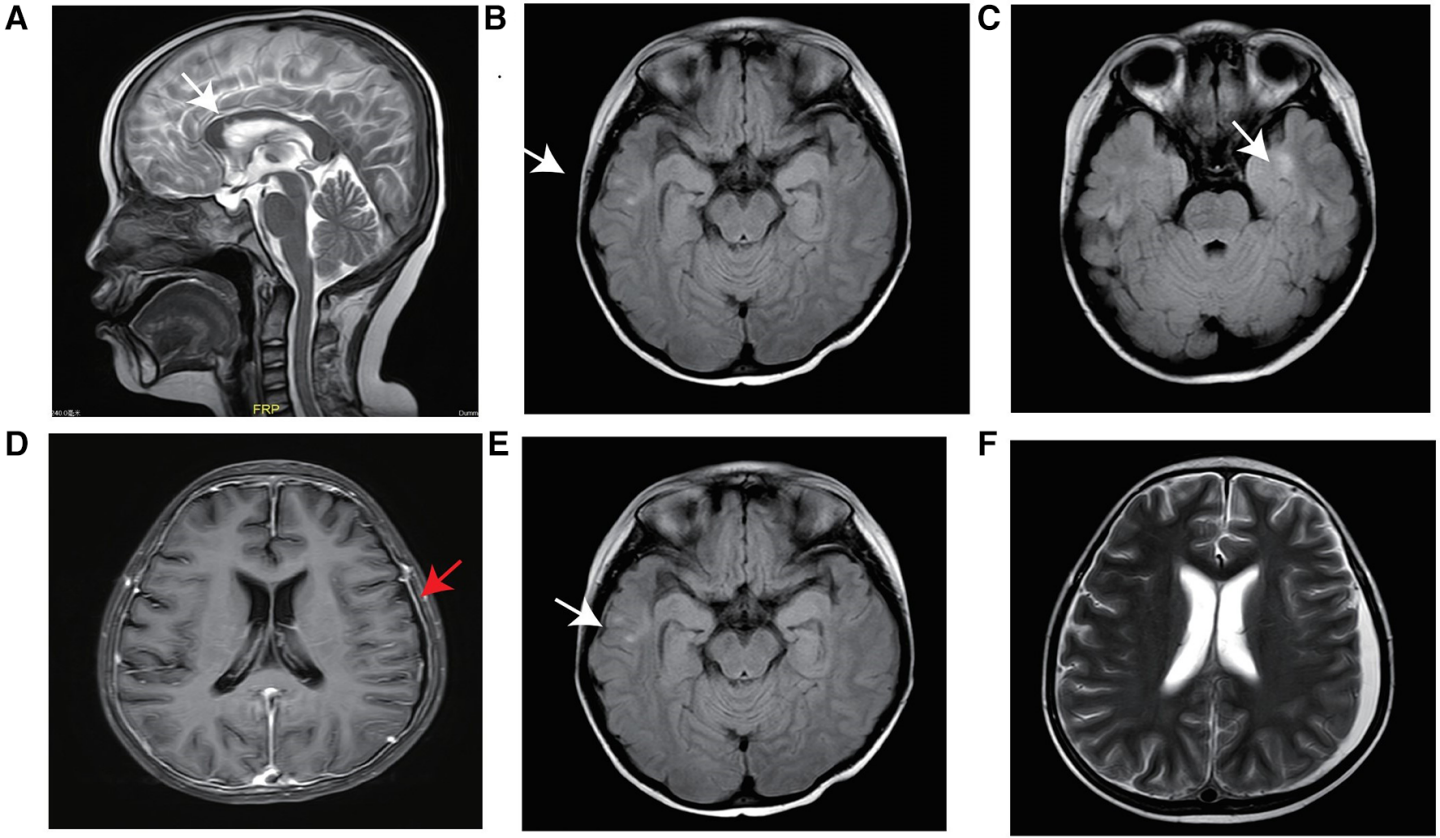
MRI of the brain. Abnormal signals from the corpus callosum body to the bilateral frontal ventricle and bilateral temporal lobe before transplantation (white arrow) (A–C), linear enhancement of the bilateral frontoparietal and occipital meninges after HSCT (red arrow) (D), abnormal signals (E), and a small amount of new subdural effusion in the left frontotemporal parietal region (F).
